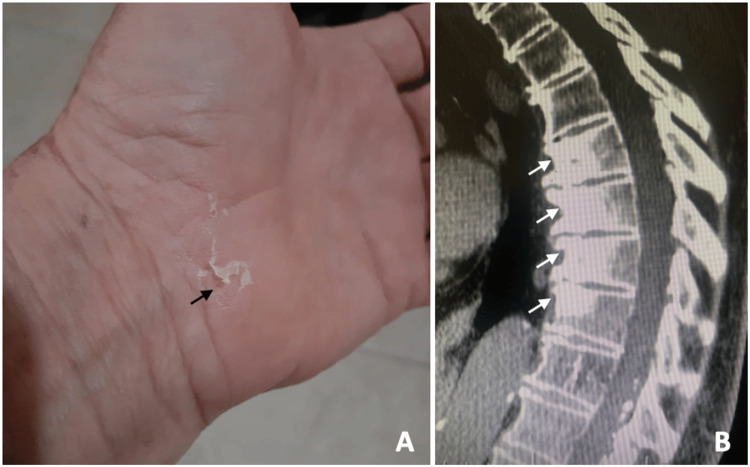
Photograph of the patient's hand demonstrating scaly phase (black arrow) (A). Sagittal CT scan showing marked sclerosis and subcortical bone irregularity, notably in the anterior half of both endplates of the T7, T8, and T9 vertebral bodies, as well as in the superior endplate of T10 (white arrows) (B).
